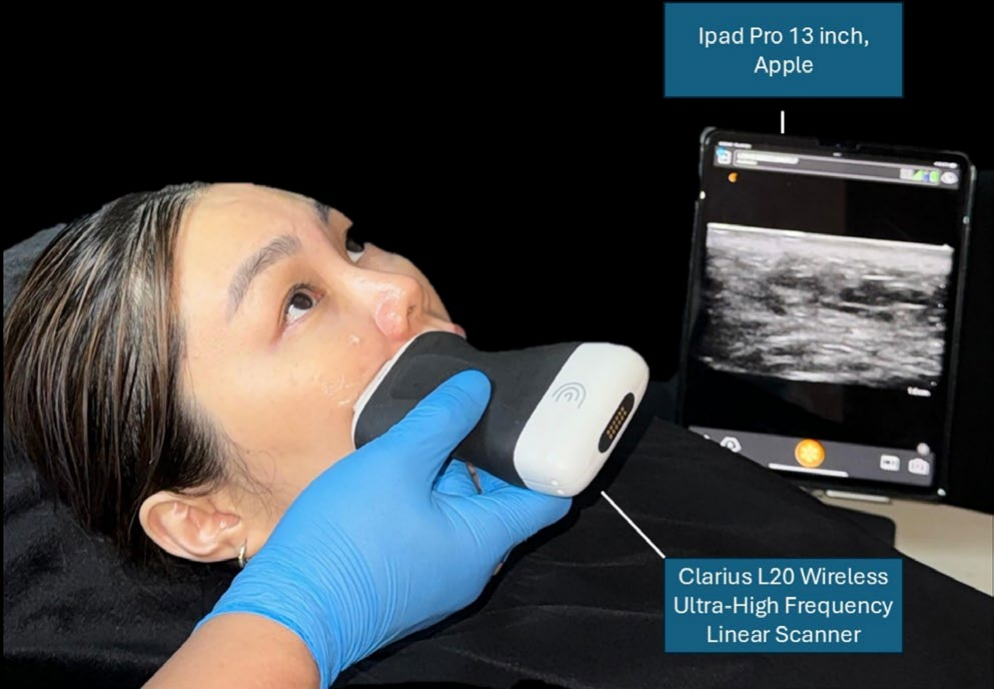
POCUS is recommended as the initial imaging modality of choice as it offers the convenience of being used in a clinic setting and the versatility of performing ultrasound‐guided treatment.
